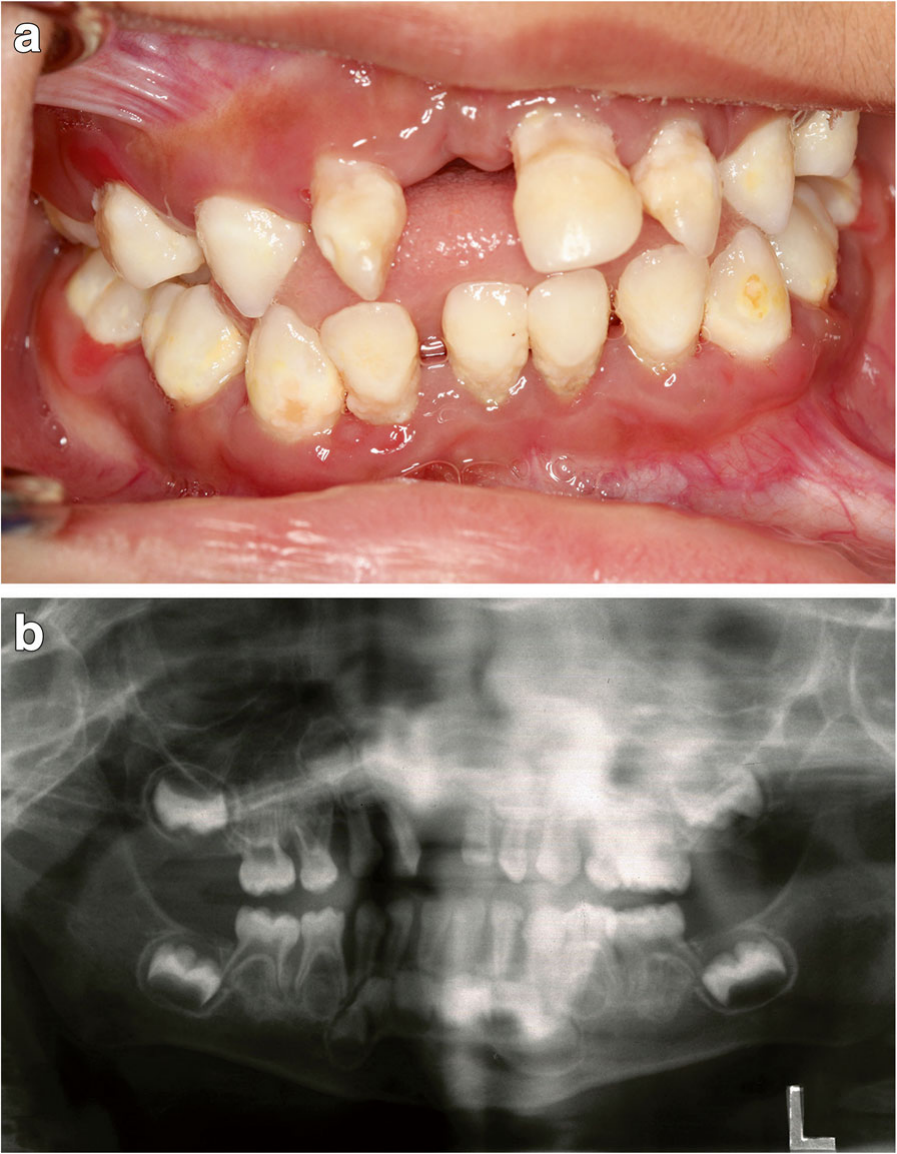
Case 2. a Intraoral appearance with gingival inflammation, plaque accumulation, migration of teeth. b Panoramic radiograph showing bone destruction and interdental spacing
